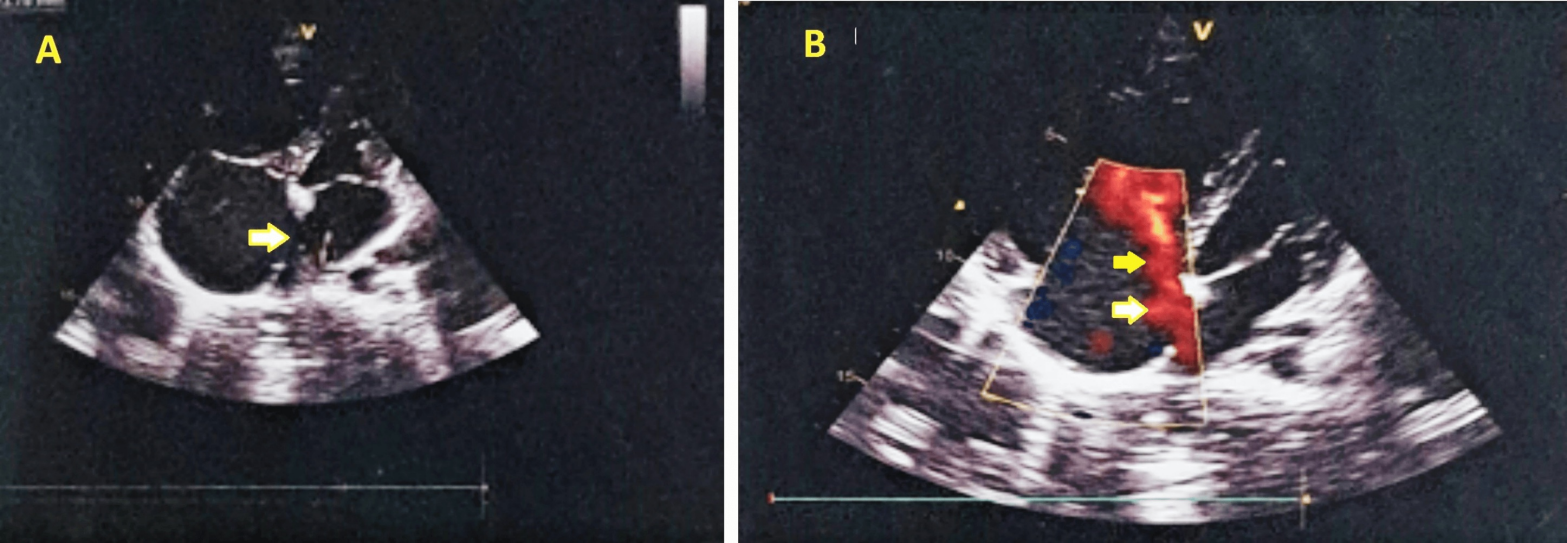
2D Echocardiography showed 25mm Ostium Primum Atrial Septal Defect, dilated Right atrium and right ventricle (A), and mild tricuspid regurgitation (B).
